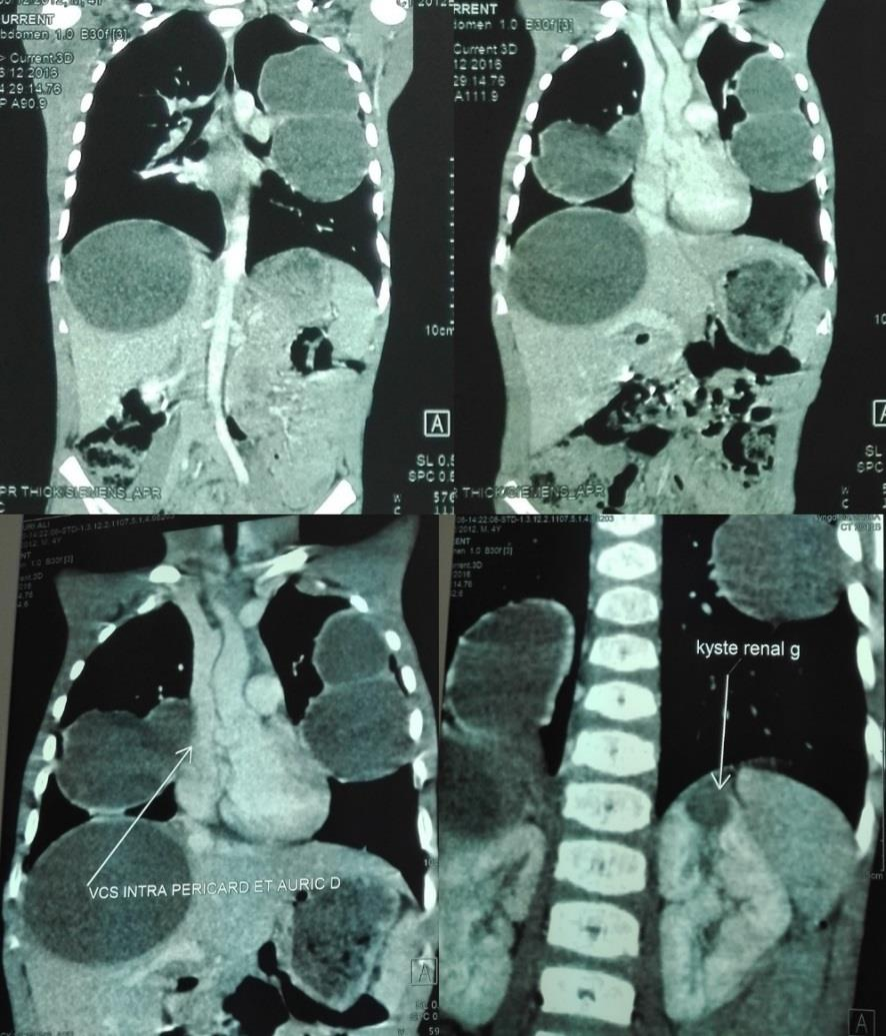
Chest and abdominal computed tomography images showing multiple cysts with involvement of the lungs, the liver and the left kidney
